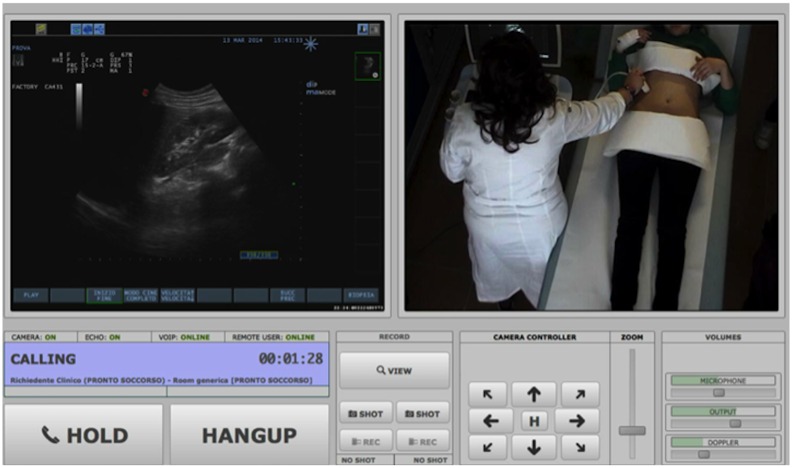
Detail of the images transmitted via tele-ultrasound, as appear on the radiologist’s screen, also showing the buttons for remote control of the webcam, zooming functions and volume.
